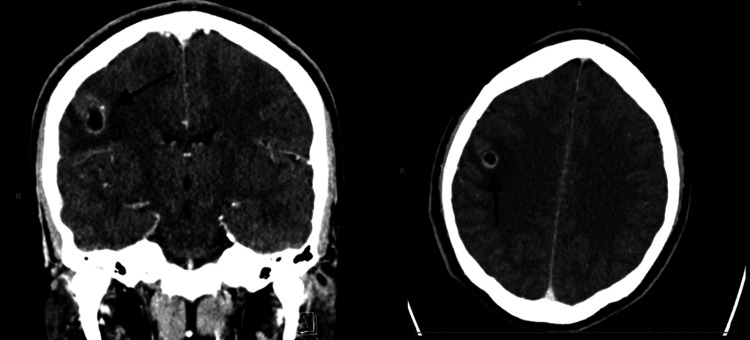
CT scan without contrast showing a solitary brain lesion indicated by the black arrow in both the coronal and axial cuts
